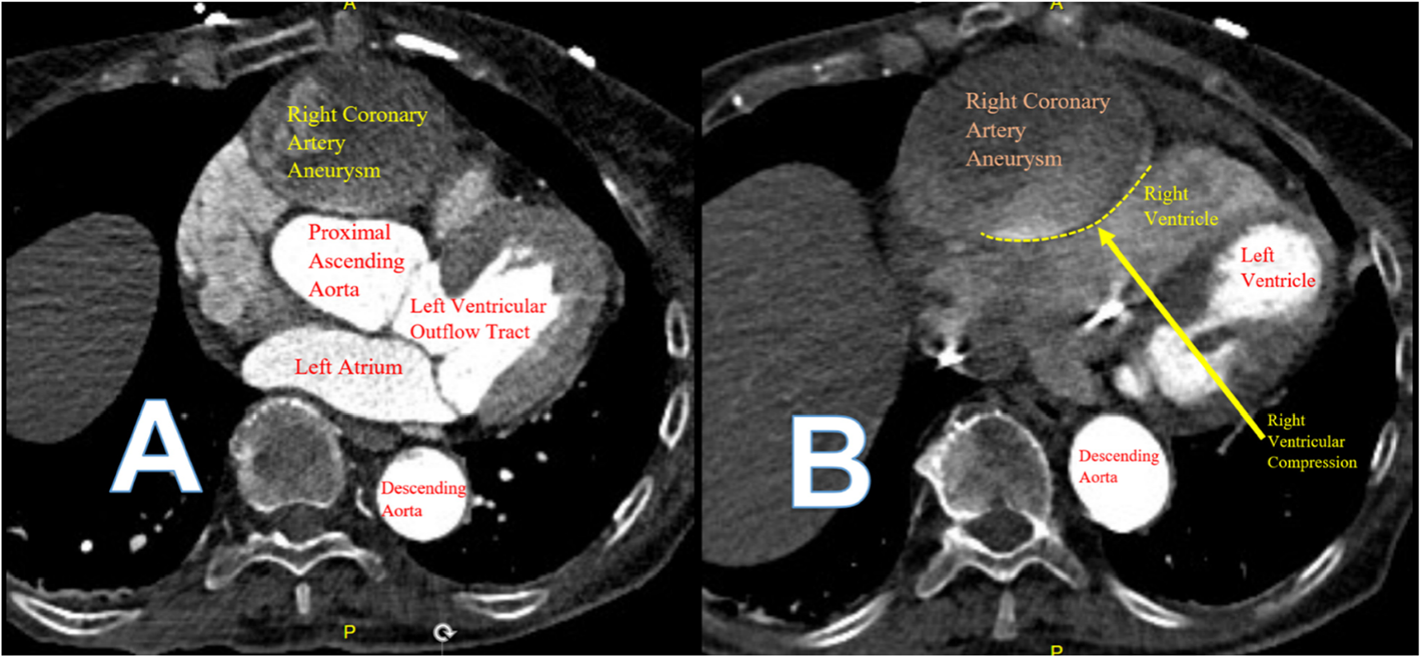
Computed Tomographic Chest Scans. A Giant RCAA at the level of the aortic root. B Giant RCAA with extrinsic compression of the right ventricle
